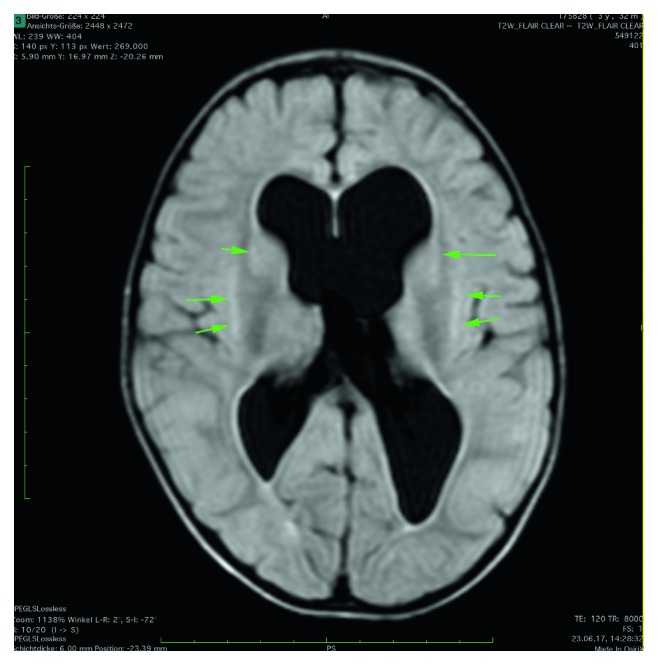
T2-FLAIR—discrete signal modulation in the corresponding T2-FLAIR sequence.
